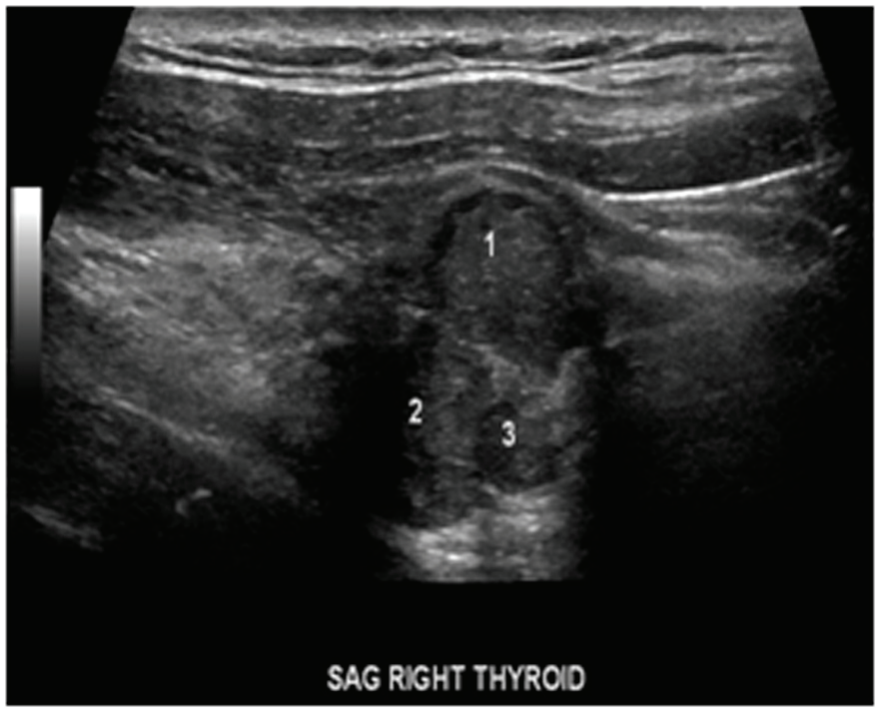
Ultrasound showing parathyroid mass in relation to the two thyroid nodules.
